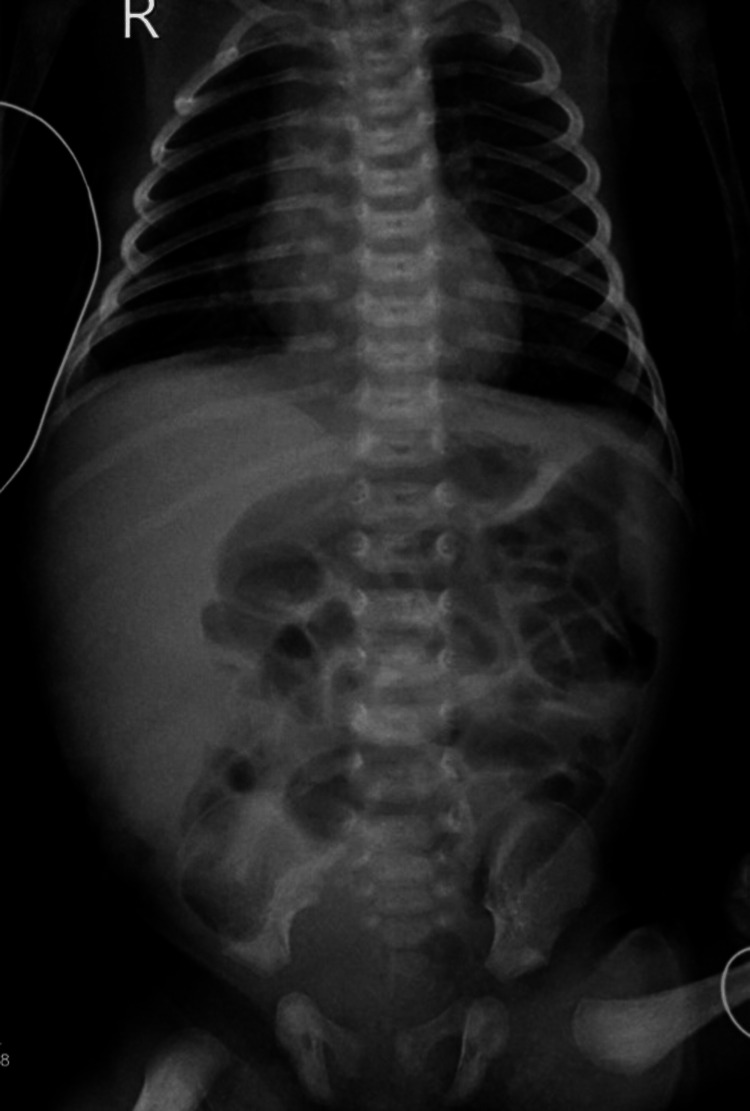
A postoperative X-ray of the abdomen showed a normal position of the bowel loops with normal opacity of the abdominal organs.
